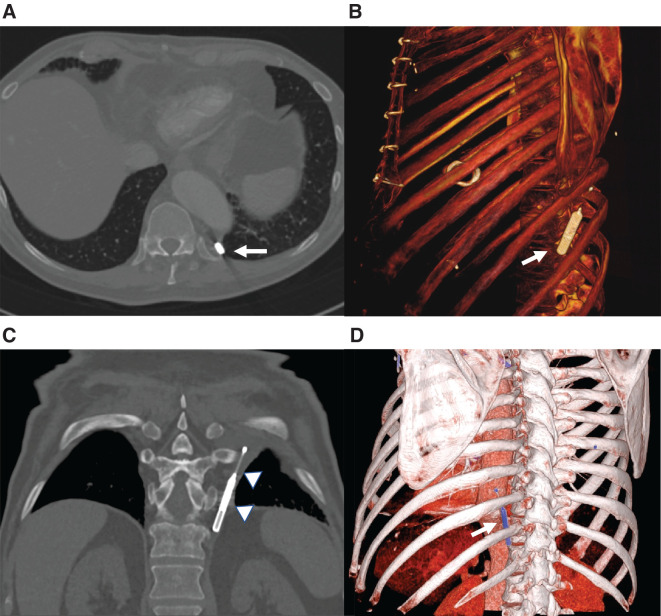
Computed tomography scan images confirming migration of the implantable loop recorder (ILR) into the left pleural cavity. A: The axial computed tomography scan shows the ILR in the posterior pleural cavity between the descending thoracic aorta and the costal skeleton (arrow). B, D: Three-dimensional volume-rendered images with color mapping provide a realistic anatomical representation of the migrated ILR (arrows) useful for surgical planning. C: Coronal oblique multiplanar reformation shows the ILR with its coil in the pleural cavity (arrowheads). No pleural effusion was present.
